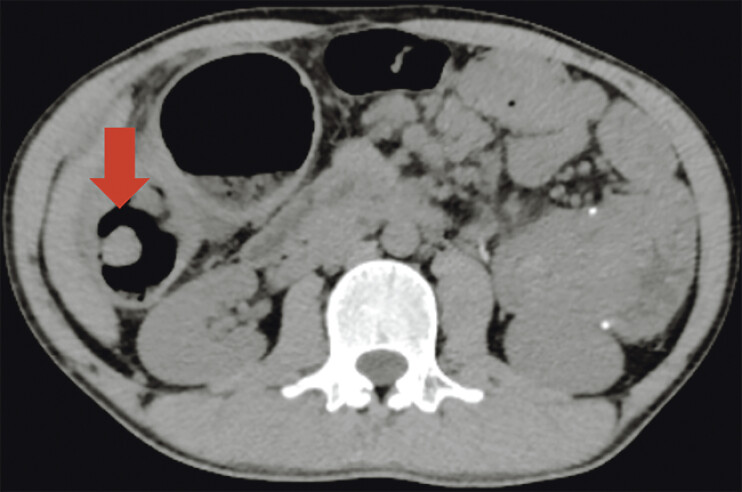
Abdominal CT showed “concentric circle sign” (arrowhead).
